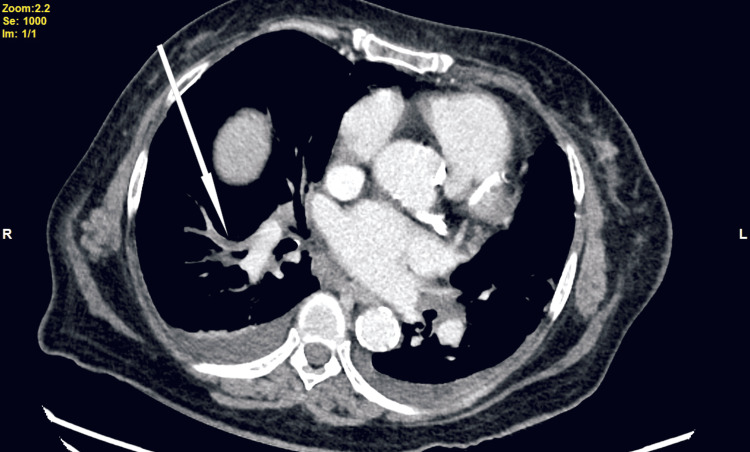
CT thorax, abdomen and pelvis, axial view (Case 3). Arrow indicates grey area of thrombus occluding a branch of the right pulmonary artery. (Small bilateral pleural effusions present.)
